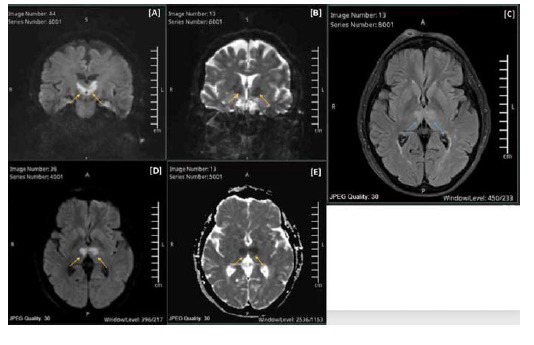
MRI head diffusion-weighted imaging (DWI). Images showing bilaterally symmetrical thalamic areas of diffusion restriction (yellow arrows) with corresponding high tbl2-weighted FLAIR signal intensity (blue arrows), which is suggestive of bilateral thalamic ischemic infarcts due to an artery of Percheron infarct.
